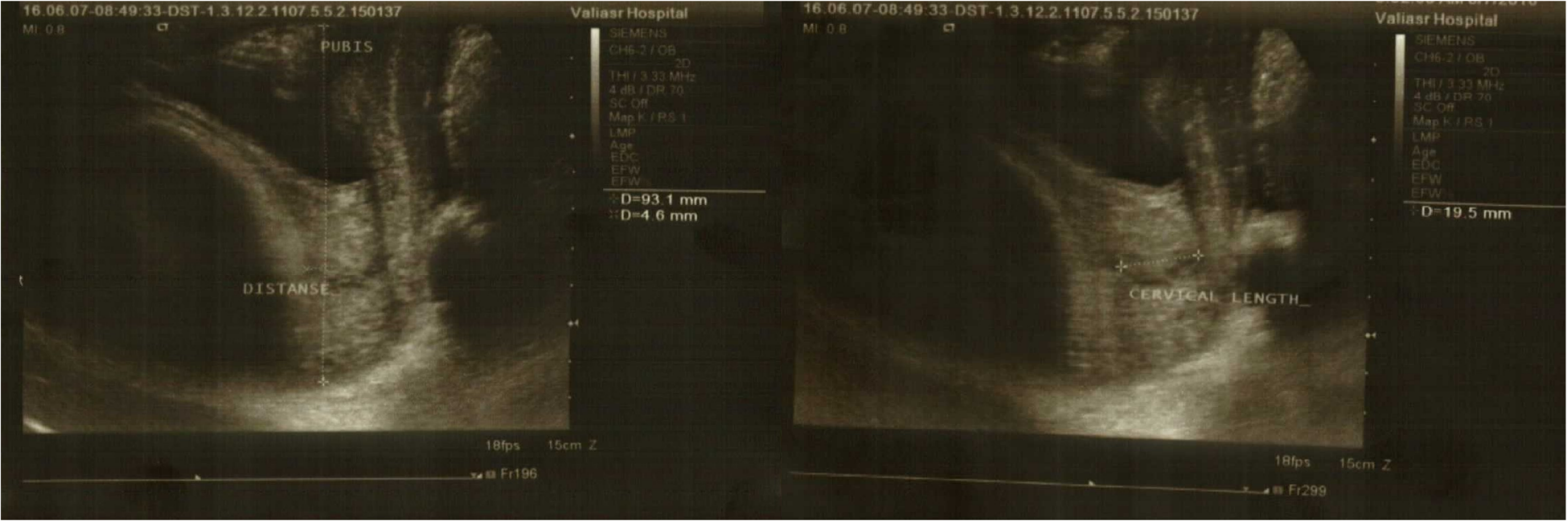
Cervical length and fetal head-symphysis pubis distance as measured in sonographic images
